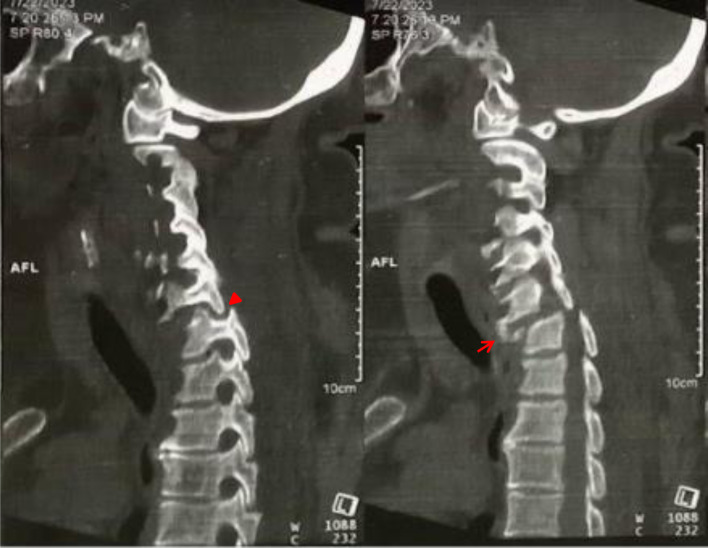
Dynamic radiographs of the cervical spine showing neglected dislocation C5–C6 treated conservatively. Note the stability of the dislocated segment and anterior auto-fusion.
